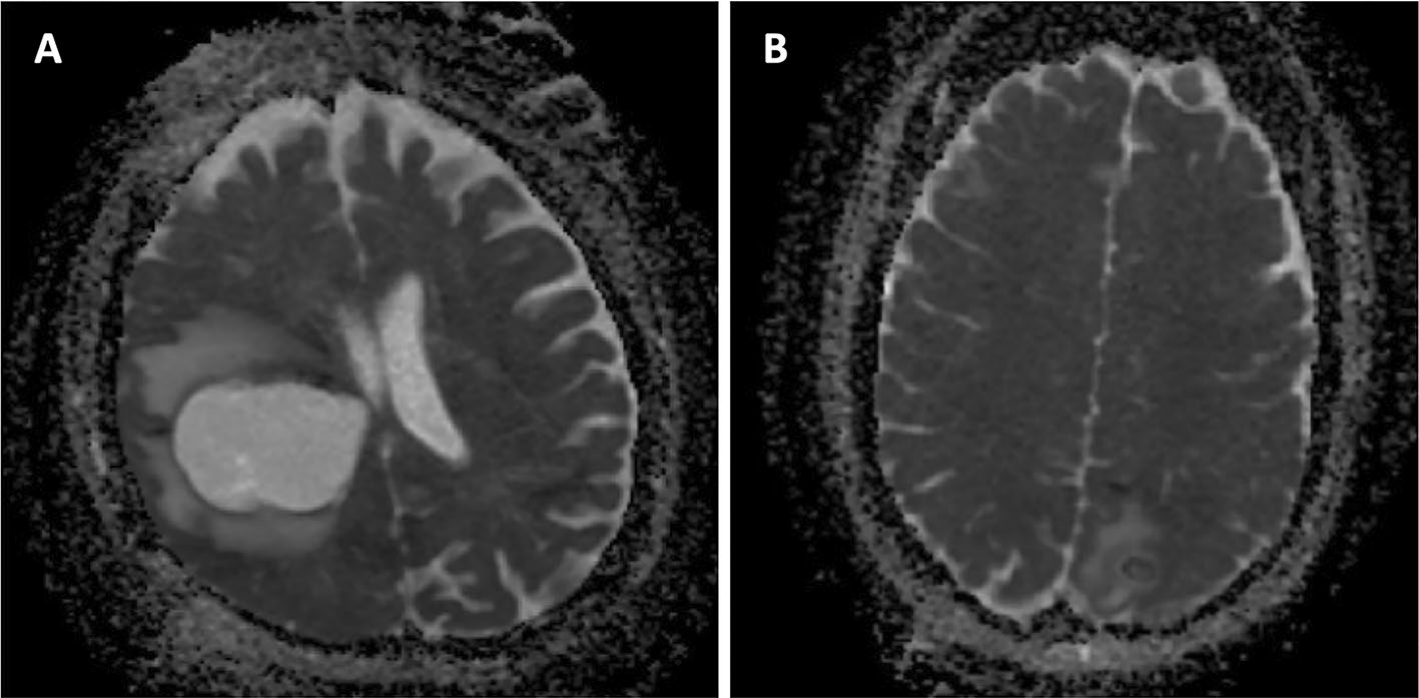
The representative images of the examined pathologies on ADC map calculated from DWI with b value 0—(A) glioblastoma, (B) abscess.
